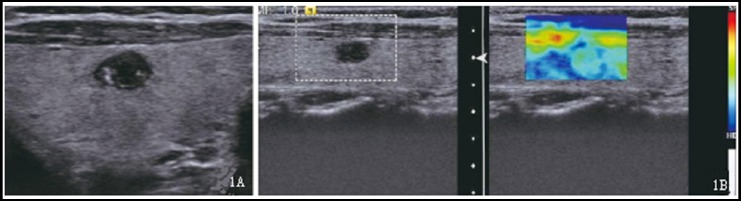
Conventional ultrasound and elastography images of nodular goiter. *Note:* Central nodules in the right lobe of the thyroid gland, 1A: conventional ultrasound examination found clear boundary (0 point), extremely low echo (2 points), and internally scattered, dotted strong echoes (2 points), and Stacul score: 4 points; 1B: grade II elastography imaging, pathological result: nodular goiter.
